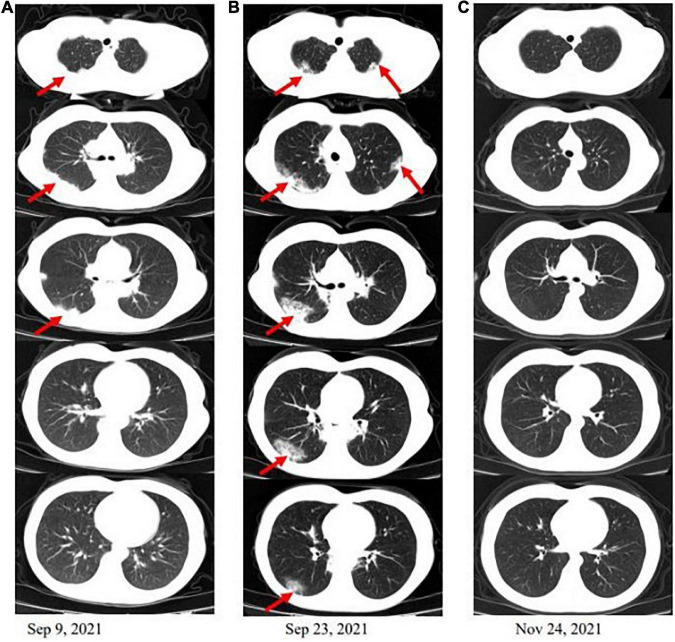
Computed tomography (CT) scan of the chest. (A) There are lesions of infiltrates under the pleura of the right lung when the patient is admitted. (B) After 2 weeks of antibiotic therapy, countercheck thoracic CT shows that pulmonary infiltrates are not significantly absorbed and appear to spread to the left lung and lower in the right. (C) The infiltrations under the pleura in both lungs were basically absorbed after a mouth of corticosteroid therapy.
